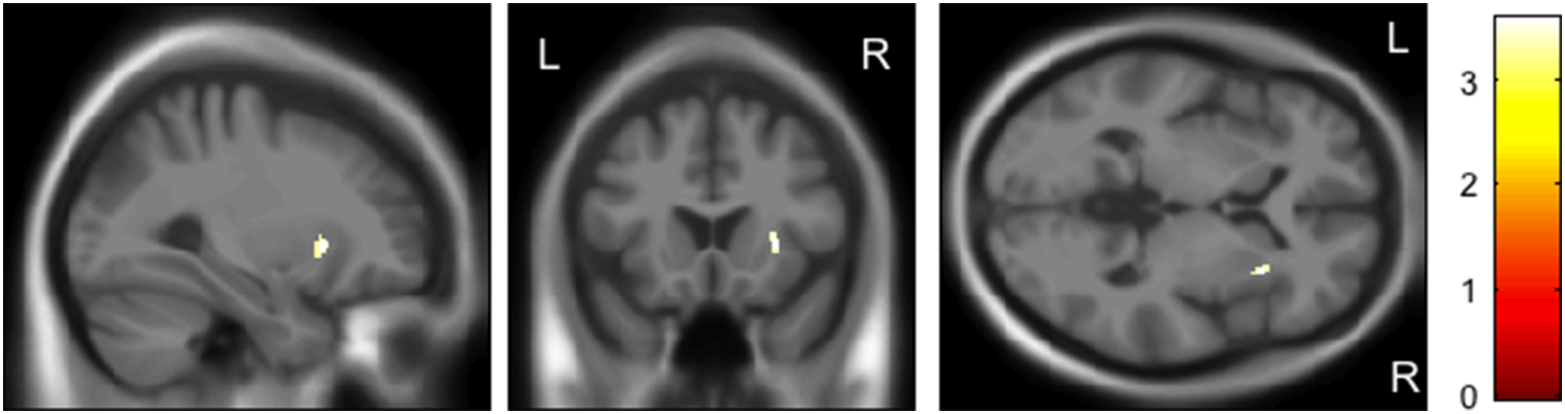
Impact of the *rs28365859* genotype on gray matter volume of the right putamen in healthy controls. The G allele homozygotes had a significantly larger right putamen than the C allele carriers. Anatomical localizations are displayed on the normal template MR images in three directions. The color bar shows t values corresponding to the color in the figure.
